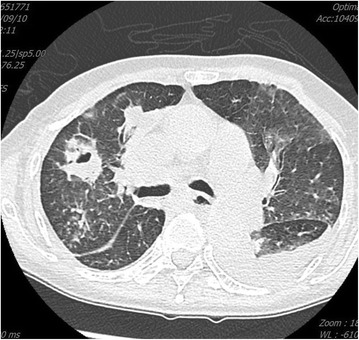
Computed tomography revealing right upper-lung pneumonia, the air–fluid level, and septic embolism
